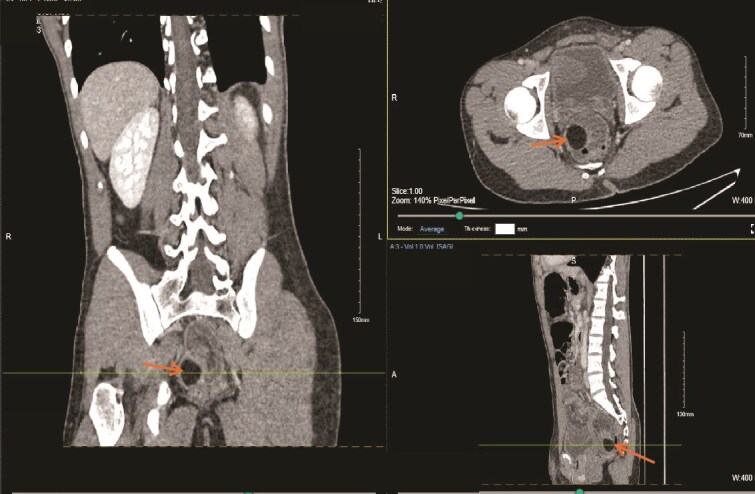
Contrast-enhanced CT scan of the abdomen demonstrating a 24 mm fat-density submucosal lesion consistent with a lipoma acting as the lead point for a 10 cm colo-colic intussusception, with associated pelvic free fluid.
